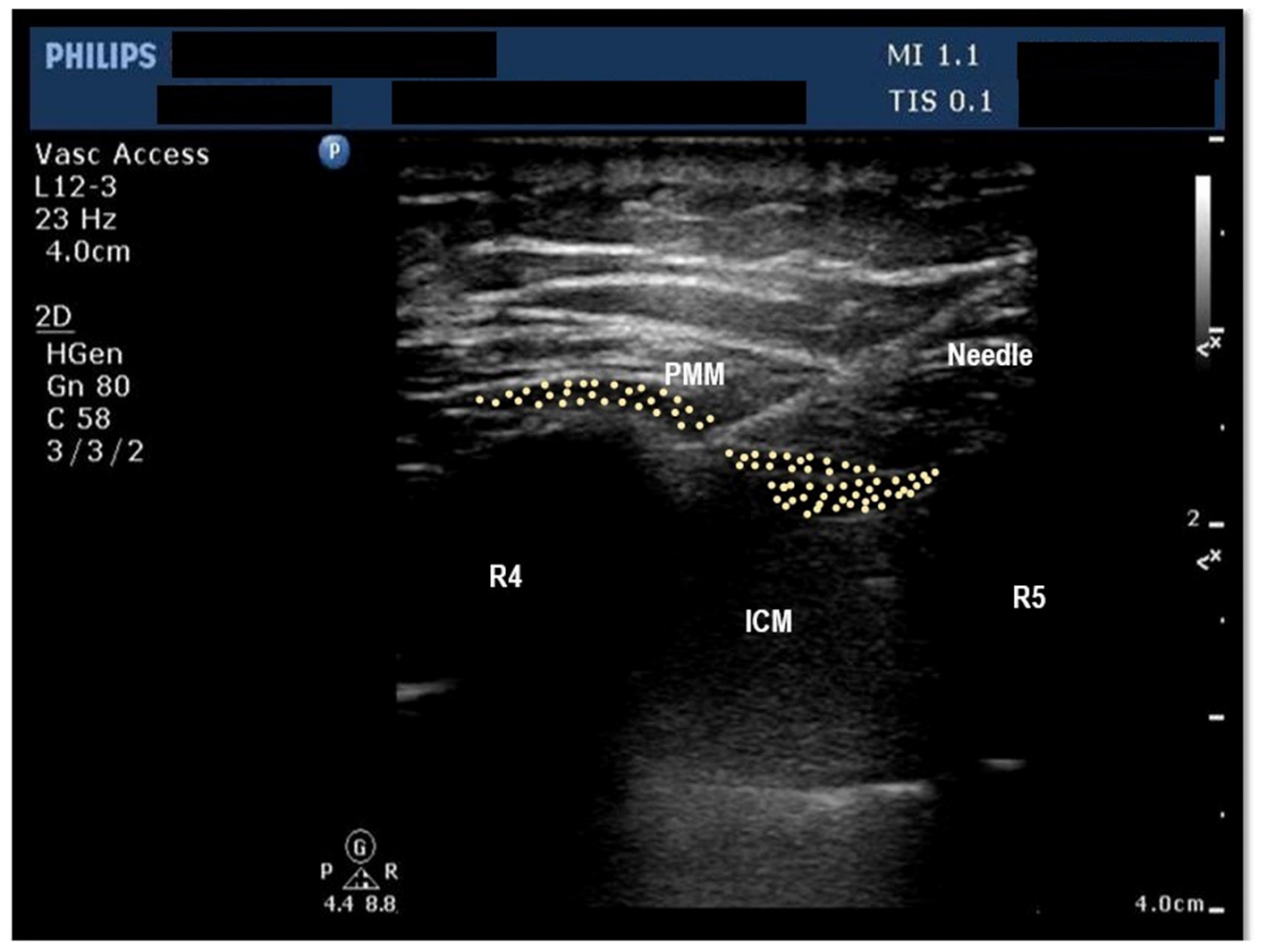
Ultrasound image of the chest wall showing anterior chest wall structures at the level of 4th Intercostal space. PMM, Pectoralis major muscle, ICM, Intercostal Muscles, R4, Fourth rib, R5, Fifth rib, Shows the position of needle, pleura and lung parenchyma.
